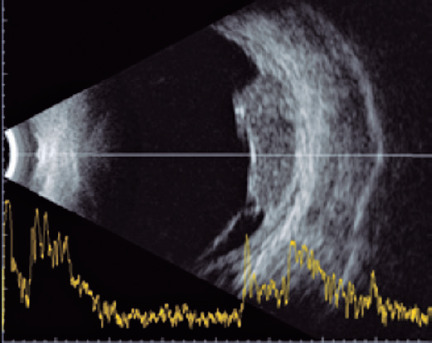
Ultrasonography image of the left eye, showing a choroidal mass
